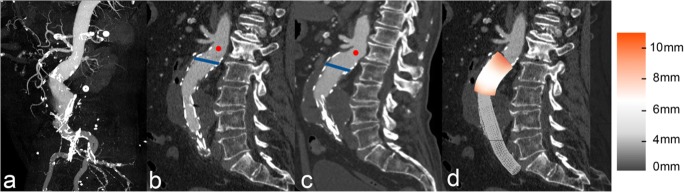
a–d 92-year-old male patient with severe angulation and large diameter (28 mm) of the infrarenal neck on pre-procedural CTA-maximum intensity-projection images (a). Comparing sagittal pre-discharge (b) and 3-year follow-up (c) CTA images, widening of the proximal aortic neck diameter and significant migration (RSD 13 mm vs 25 mm) were observed. The red dot (b, c) marks the lowest renal artery, while the blue line represents fabric markers of the stent-graft coverage. Significant stent-graft surface movement (> 9 mm) occurred at the proximal landing zone, visualized by 3D matched surface models of postprocedural and final follow-up CTA (d)
